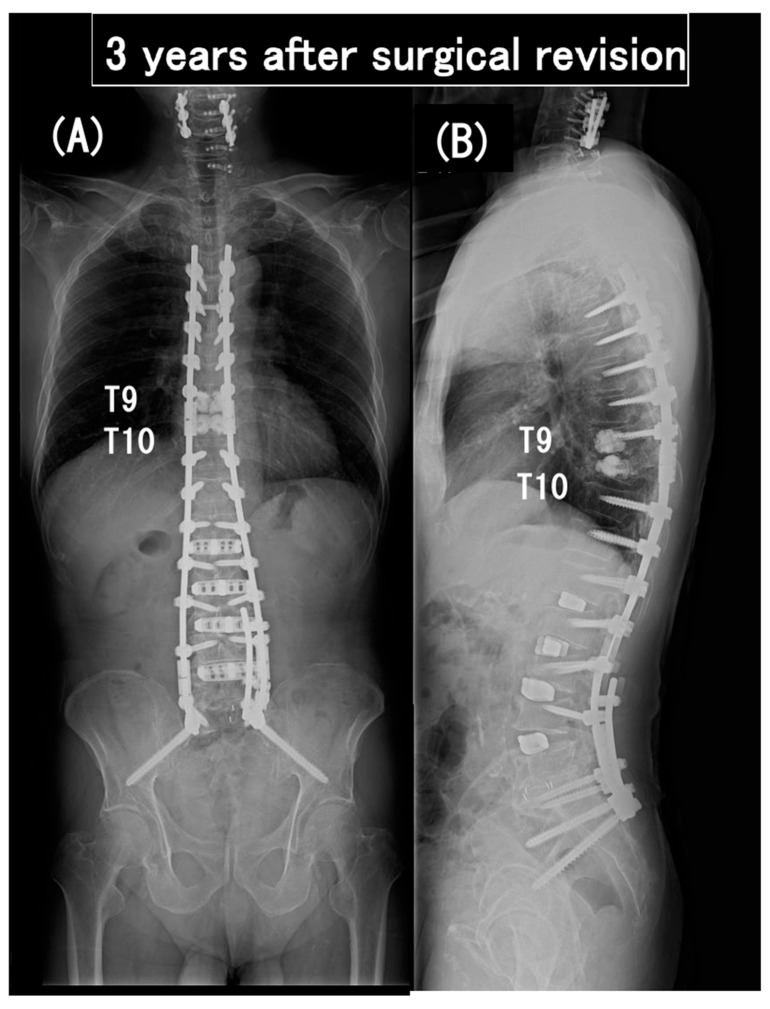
Coronal (A) and sagittal (B) full-length standing radiographs obtained 3 years after surgical revision. The paraparesis gradually improved from modified Frankel grade C to D2.
